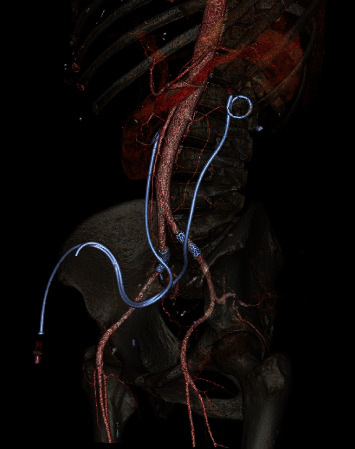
Three-dimensional abdominal CT scan that demonstrated bilateral iliac artery endoprosthesis and ureteral stents (sagittal evaluation).
